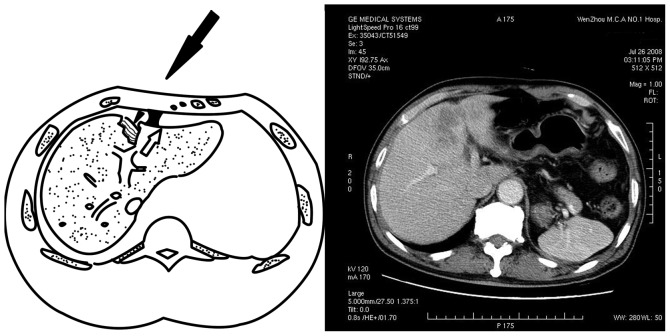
Acceleration injury in the left lobe of the liver. When the blunt force (black arrowhead) pushes the left lobe of the liver toward the back, the falciform ligament serves as a counterforce (empty arrow). The section of the liver that bears the shearing stress moves posteriorly and is lacerated. CT film shows a hepatic injury caused in this way by a traffic accident.
